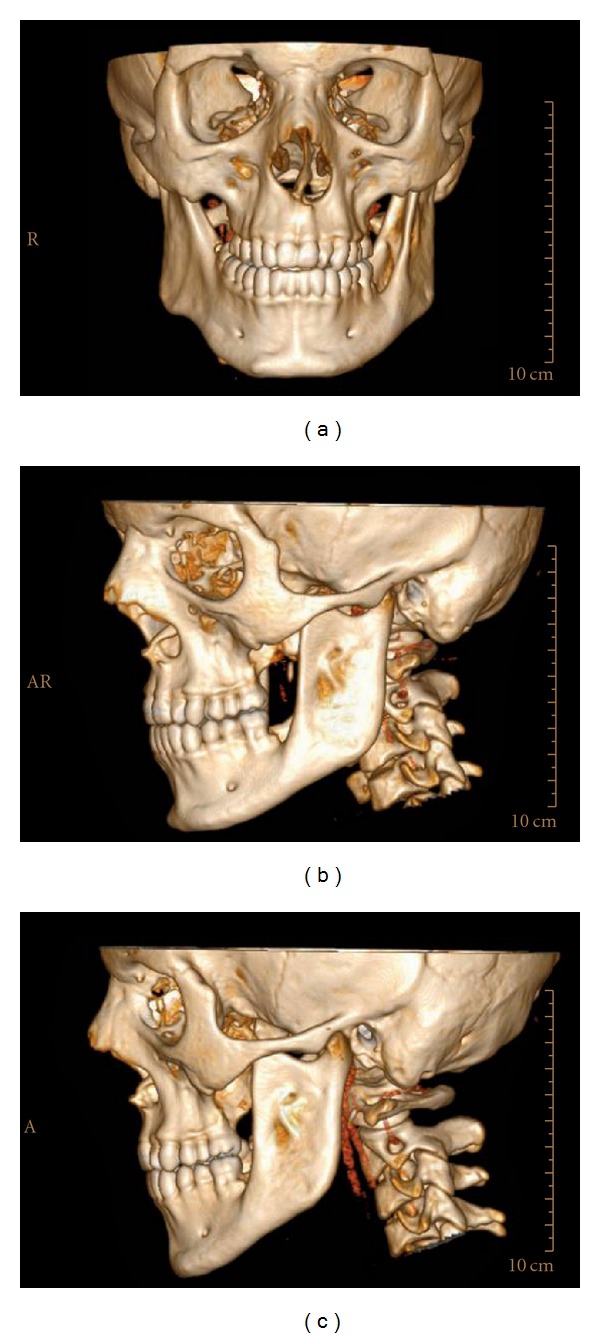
3D CT frontal (a) sagittal (b,c) images demonstrates the disappearance of a multilocular bony defect after 1 year follow up.
